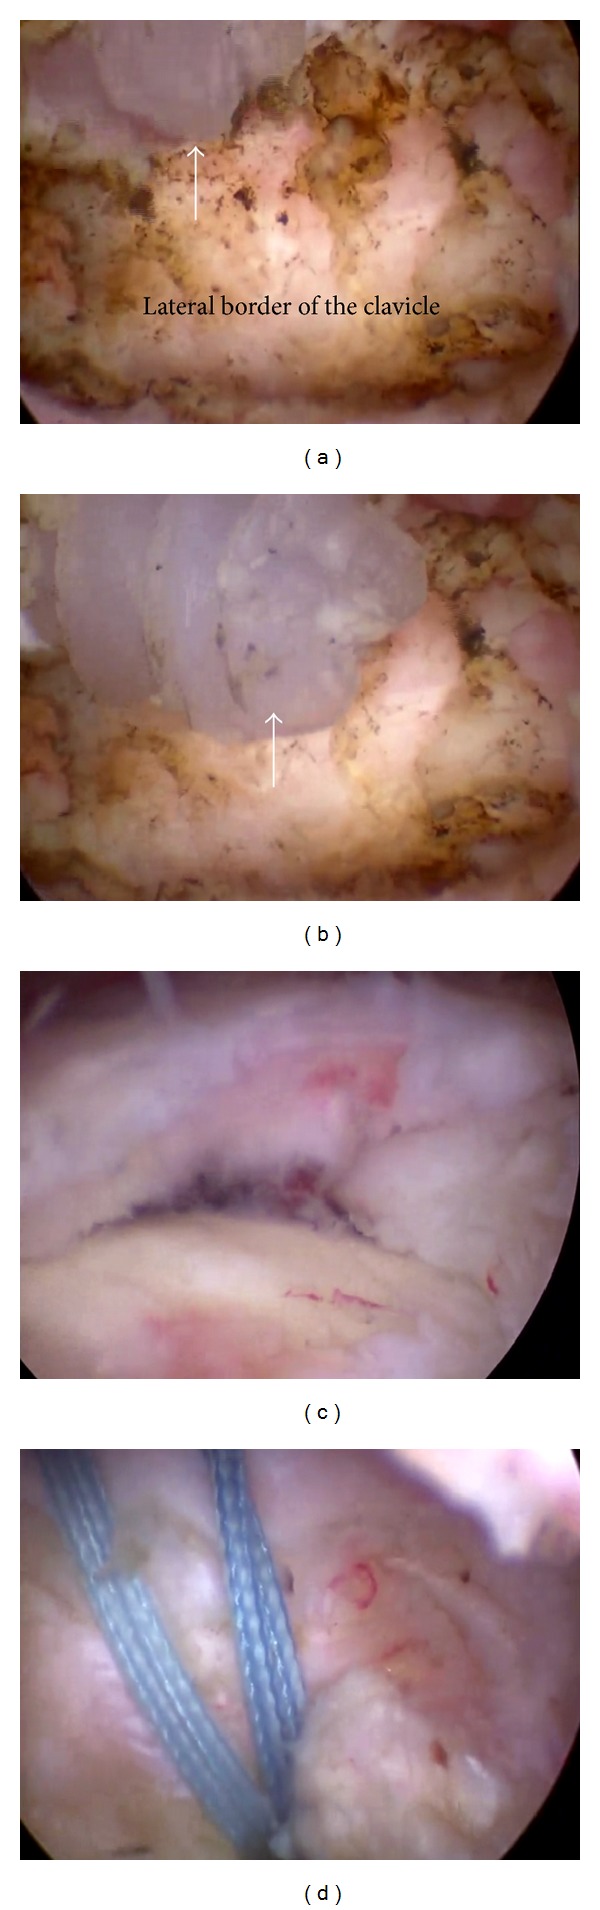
Arthroscopic images confirmed the MRI findings. (a) View through the posterior portal: it is able to see the bioabsorbable anchor in the ACJ (white arrow). We used a spinal needle inserted through the skin superiorly through the ACJ joint, to push the anchor down ((b) white arrow) and retrieve it from the lateral working portal. (c) The retear of the rotator cuff was repaired with double row technique (d) using 2 medial and 1 lateral bioabsorbable anchors.
